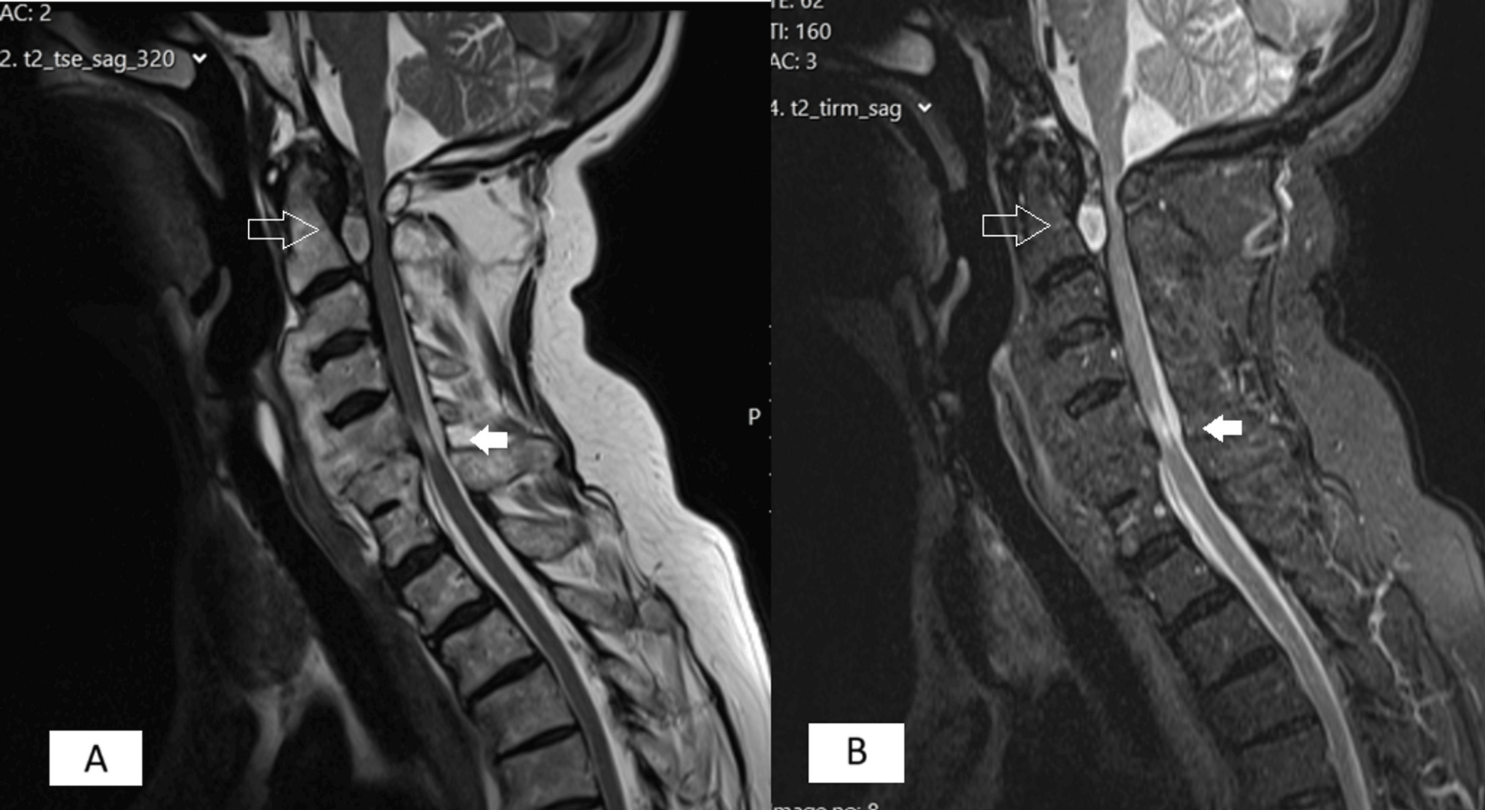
MRI before surgery Unfilled arrow shows a retro-odontoid inflammatory mass with stenosis of the vertebral canal at C1-C2 and filled arrow shows a multilevel discopathy with degenerative changes causing stenosis at C5-C6 level. (A) T2-weighted image. (B) short tau inversion recovery (STIR) image
